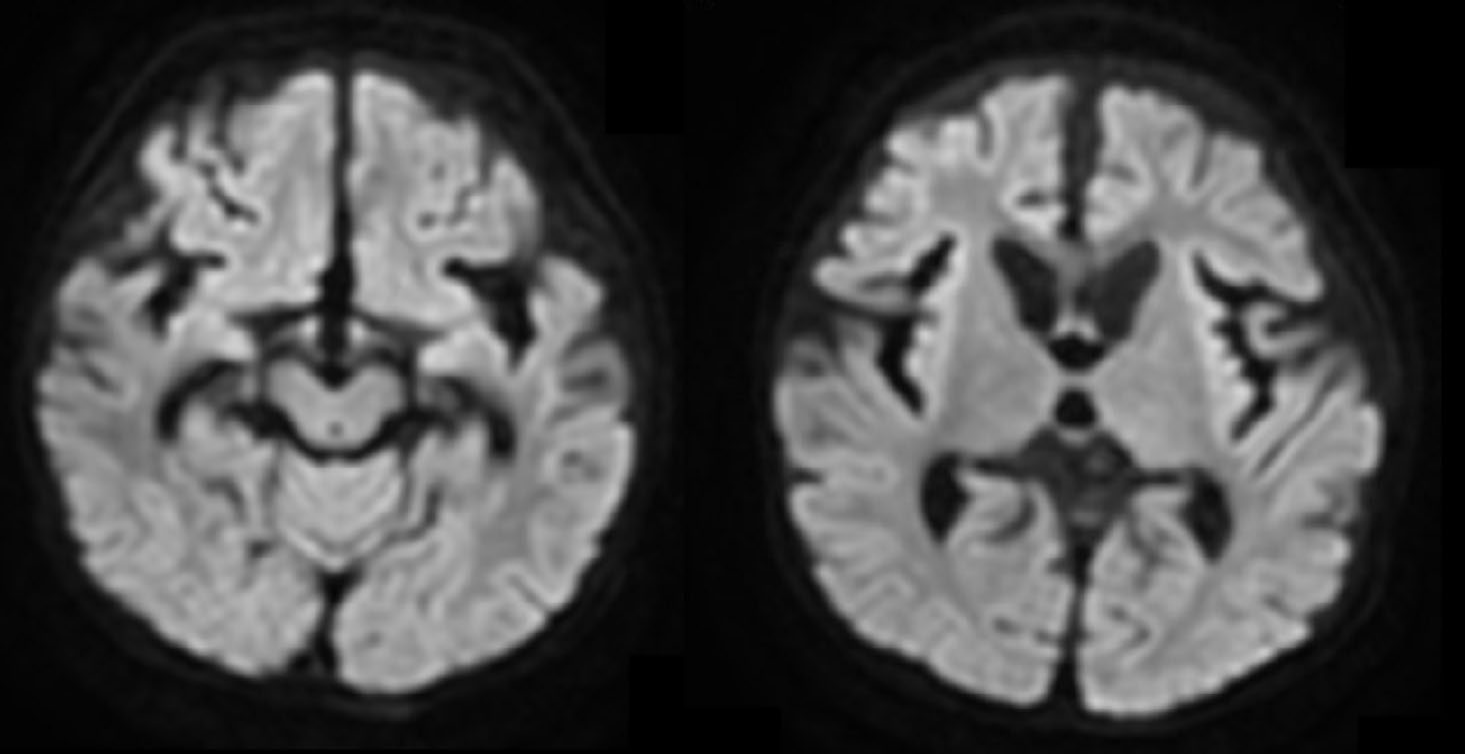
MRI on Day 88 after Admission. Cerebral parenchymal atrophy was observed on day 88 post‐admission. The abnormal signals observed in the bilateral globus pallidus and substantia nigra on diffusion‐weighted imaging have improved.
